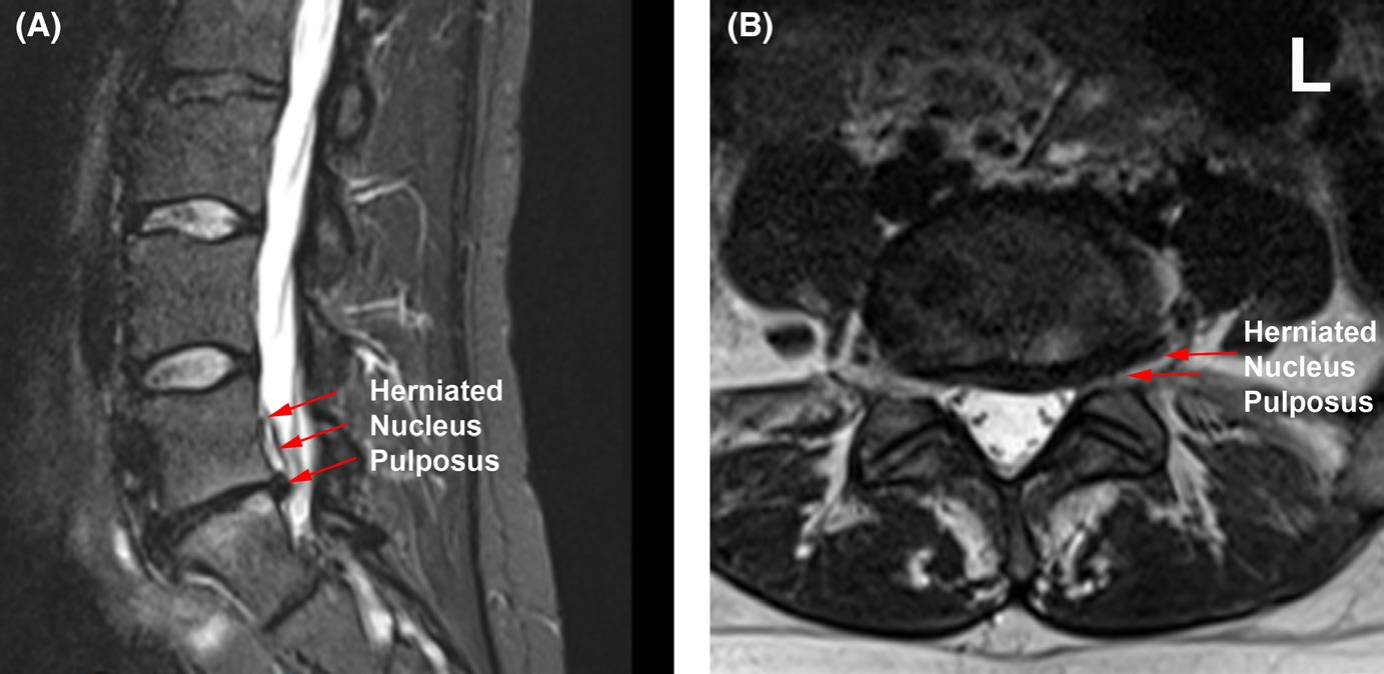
Lumbar spine MRI without contrast showing a disk herniation at L5‐S1 level. A, Sagittal MRI: T2‐weighted STIR (Short‐TI Inversion Recovery), B, axial MRI: T2‐weighted
